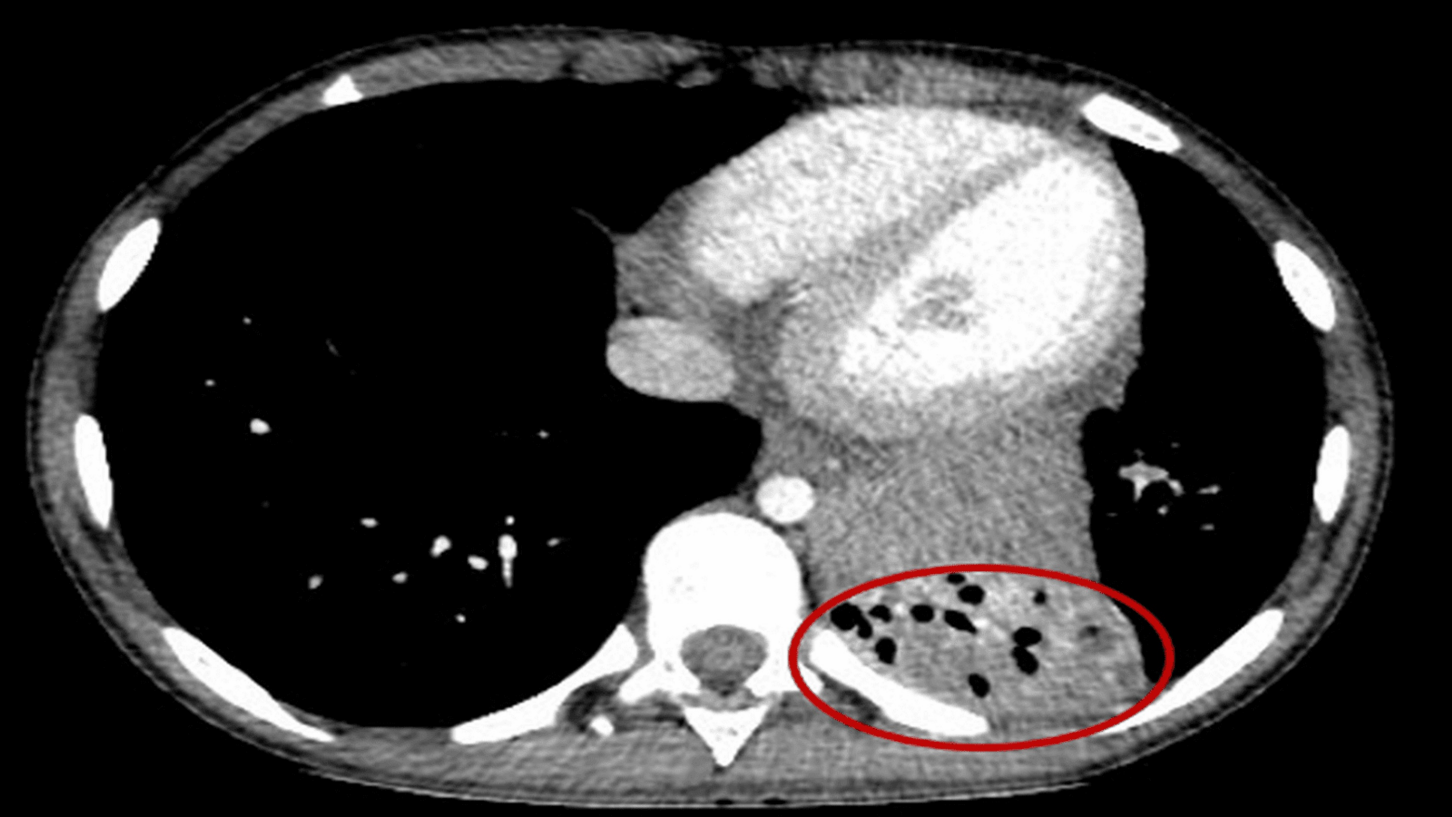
High-resolution chest CT (axial view) showing left lower lobe collapse with cystic bronchiectasis and calcified hilar lymphadenopathy. CT: computed tomography
